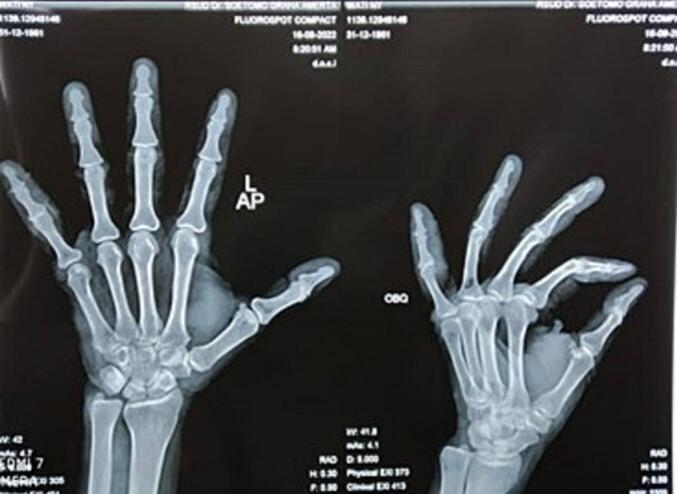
Plain radiograph of the left wrist with (a) posteroanterior and (b) oblique view post operation.
